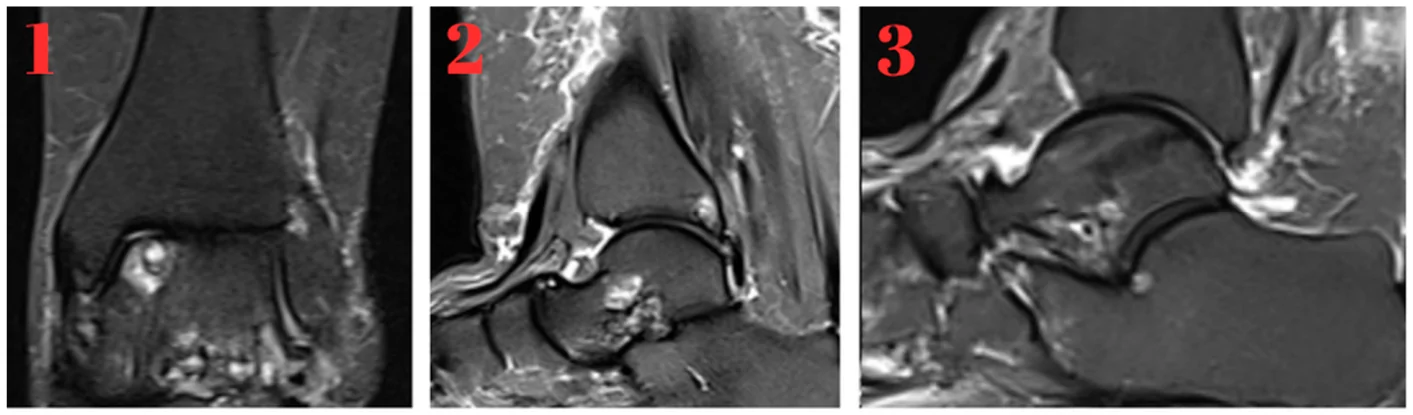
Representative MRI findings in the ipsilateral ankle. (1): osteochondral finding of the talus (coronal T2-weighted image); (2): osteochondral finding of the distal tibia (sagittal T2-weighted image); (3): subcortical cyst at the subtalar joint (sagittal T2-weighted image).
